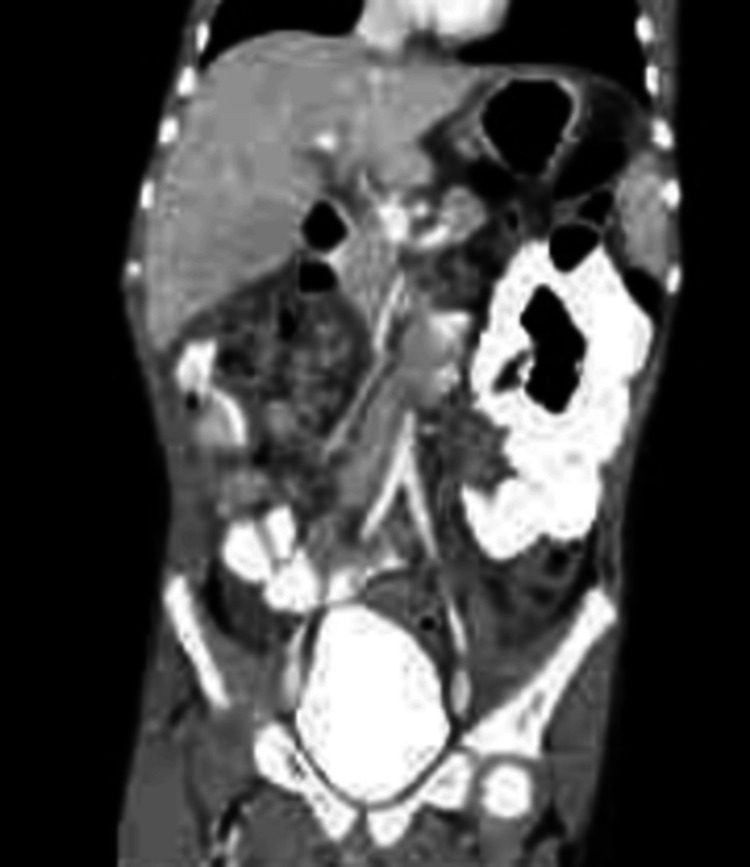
Computed tomography (CT) with oral and IV contrast demonstrating dilated loops of bowel
